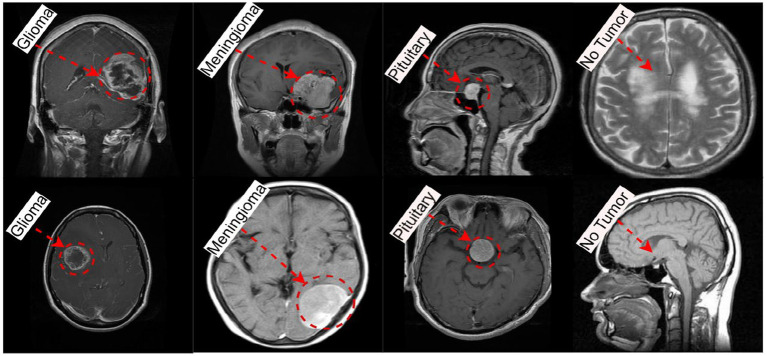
Sample in the data brain tumor MRI dataset and MRI for brain tumor with bounding boxes.
